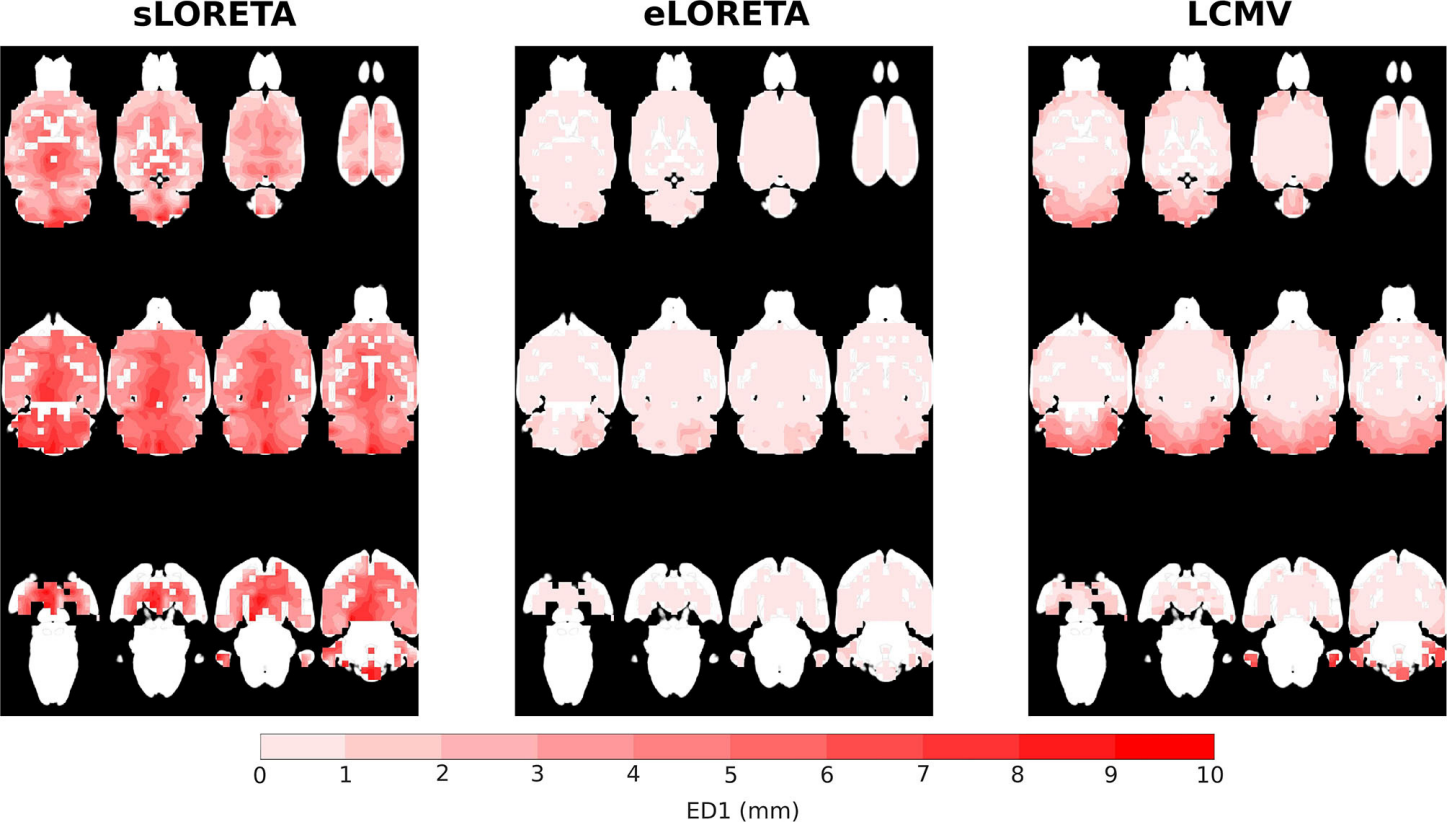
ED1 values interpolated on MRI scan axial slices for sLORETA, eLORETA, and LCMV inverse solvers with SNR 25 dB.
